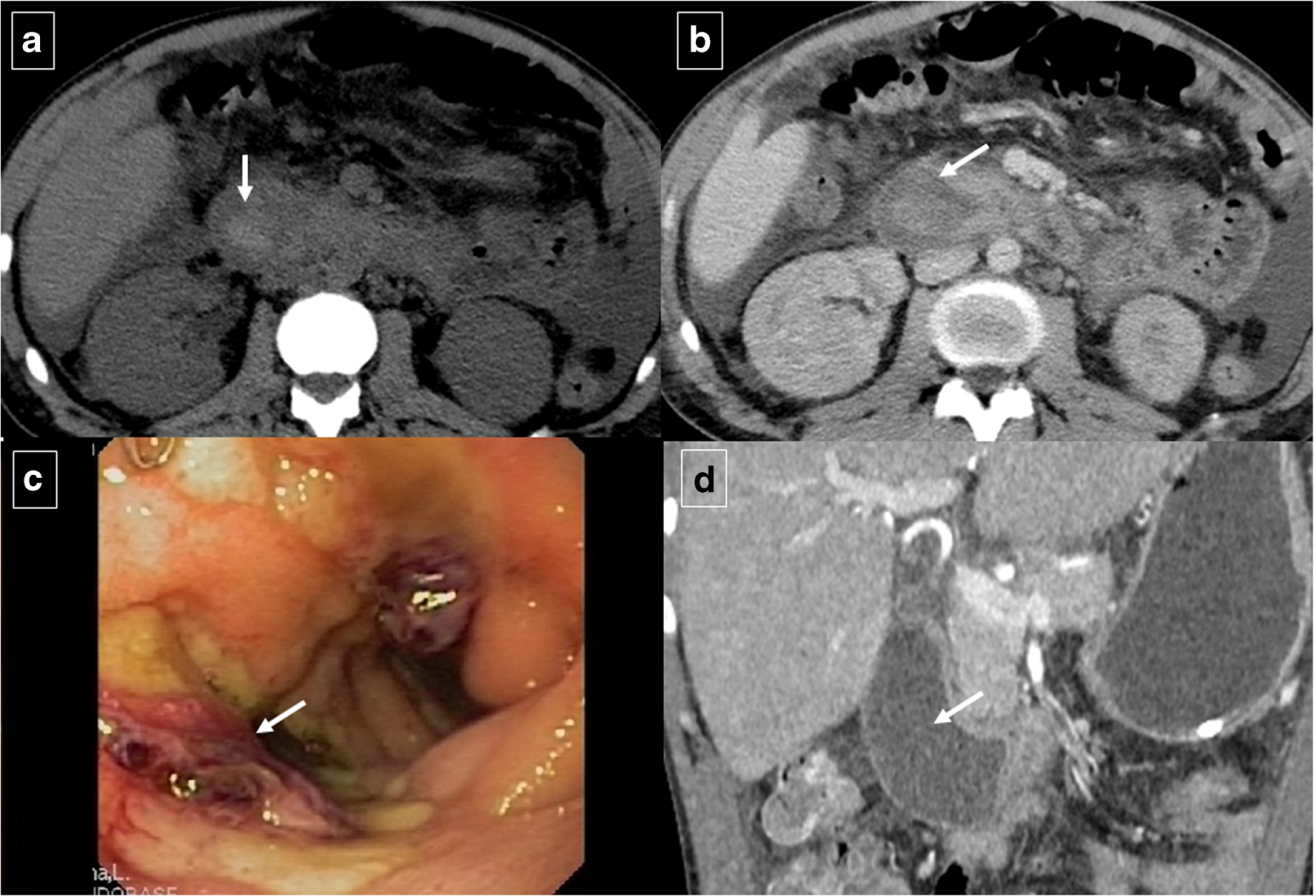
Intramural duodenal hematoma in a 28-year-old man with a history of cocaine abuse. a Unenhanced CT shows a spontaneous hyperdense thickening of the duodenal wall (arrow). b Axial contrast-enhanced CT shows a heterogeneous and intramural duodenal masslike appearance (arrow). c Detail of the endoscopic study in the initial diagnostic. d Reformatted coronal contrast-enhanced CT image of the same patient a few days before show a predominant hypodense expansive masslike appearance (arrow) causing a mass effect of the duodenal lumen with gastric dilation
